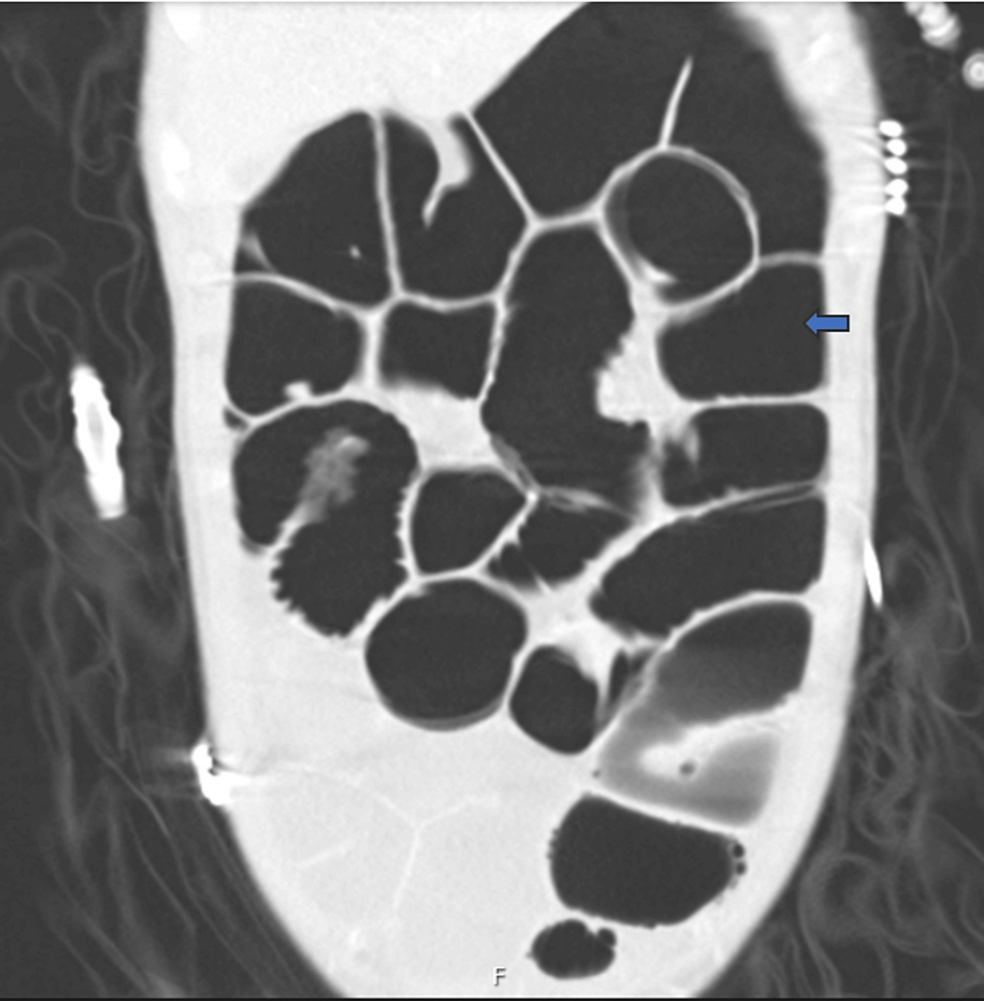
Coronal section of a CT abdomen study revealing the finding of dilated small and large bowel loops in the patient (assessed as ileus)
